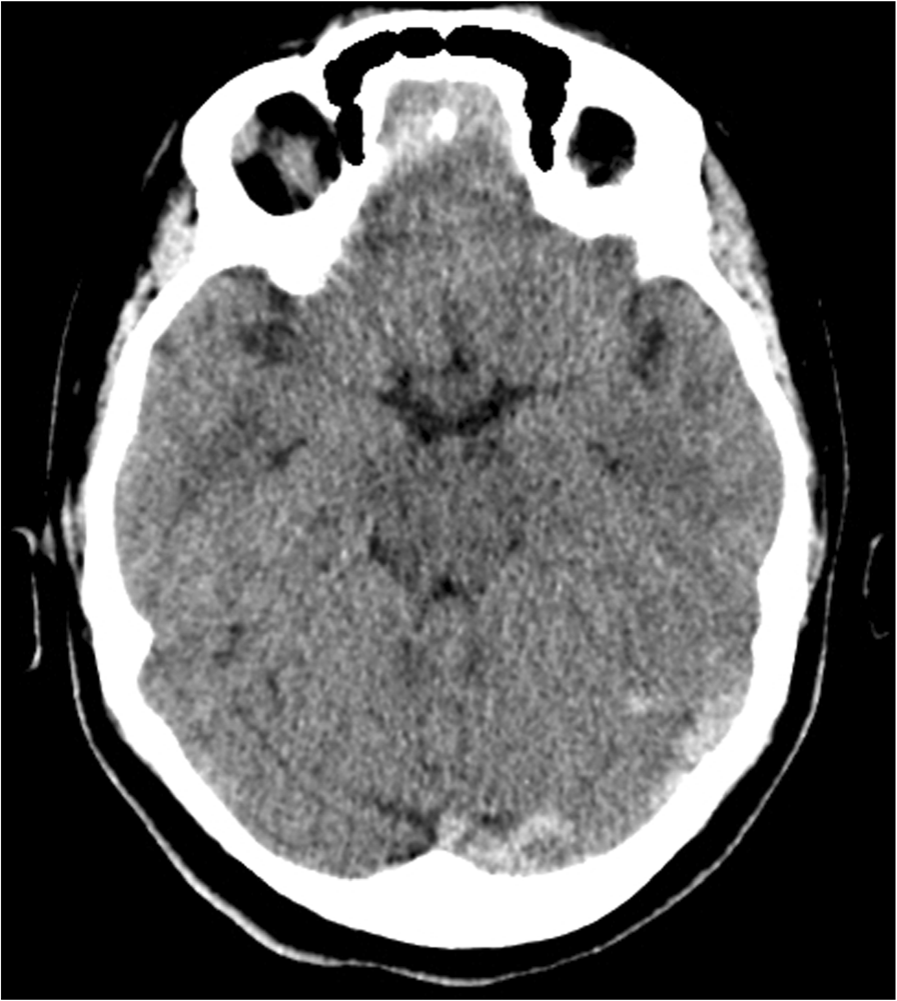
A cross sectional view of a computed tomography brain scan without contrast shows hyperdensity in the thrombosed sinus
